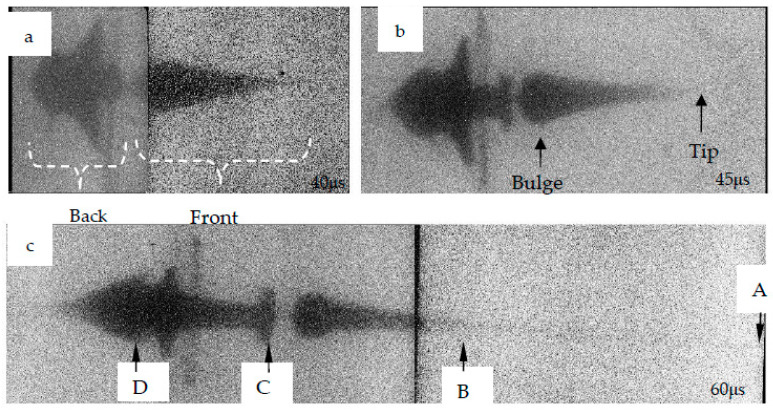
Jet image under pulsed X-ray: (a) 40 μs; (b) 45 μs; (c) 60 μs, “A, B, C, D” is the reference position.
